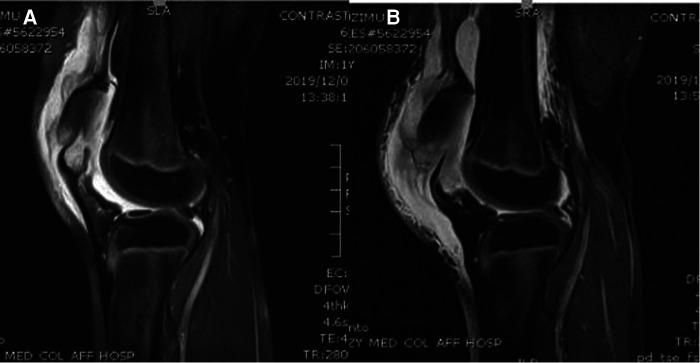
(A) Sagittal T2 MRI of the right knee. (B) Sagittal T2 MRI of the left knee.
